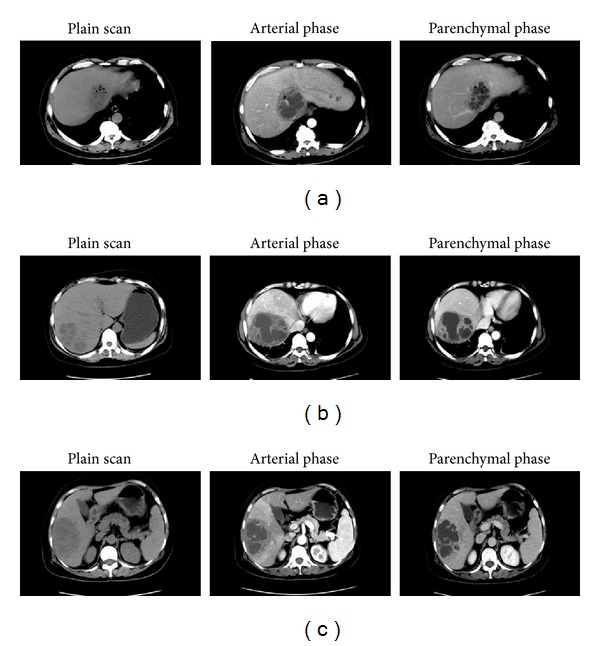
Comparison of abdominal CT images between KLA and Non-KLA.  (a) CT images of a 57-year-old male KLA patient with concomitant diabetes mellitus: circular shadow of low and uneven density can be seen in the caudate lobe near the second hepatic portal. With a diameter of 90 mm, a shadow of much lower density and gas cavities can be seen in the center of the abscess. During enhanced scanning, the margin and internal septations of abscess show a honeycomb-like structure. Intrahepatic bile ducts show slight dilation. (b) CT images of a 51-year-old female patient with *E. coli* liver abscess: irregular low-density lesion with a honeycomb-like structure can be seen in the right lobe of the liver. Obvious cystic wall enhancement can be seen during enhanced scanning. There is no stenosis or filling defect of hepatic vessels. (c) CT images of a 65-year-old female patient with *Pseudomonas aeruginosa* liver abscess: patchy shadow of low-and-even density and clear edge can be seen in the right lobe of the liver. By enhanced CT scan, the peripheral enhancement is more dramatic than the nonperipheral enhancement. Septation is visible inside the abscess, and hepatic blood vessels are evenly distributed. KLA: *Klebsiella pneumoniae* liver abscess; Non-KLA: *non-Klebsiella pneumonia*-induced pyogenic liver abscess.
